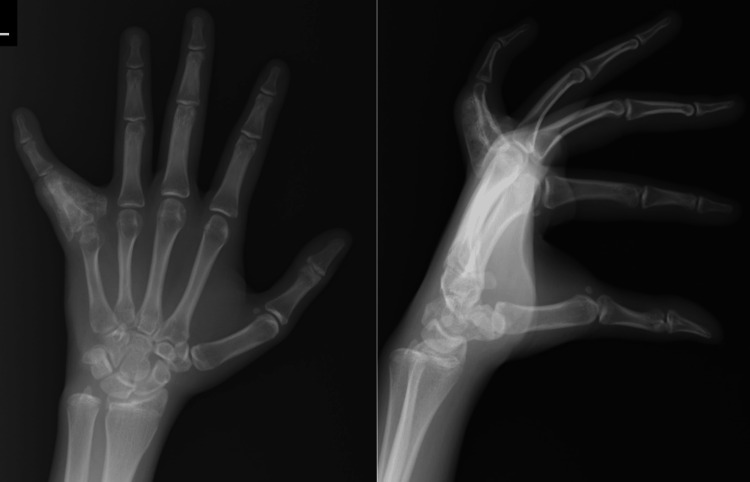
Anteroposterior and lateral view radiographs of the left hand show a large periosteal reaction on the proximal phalanx of the left little finger.
